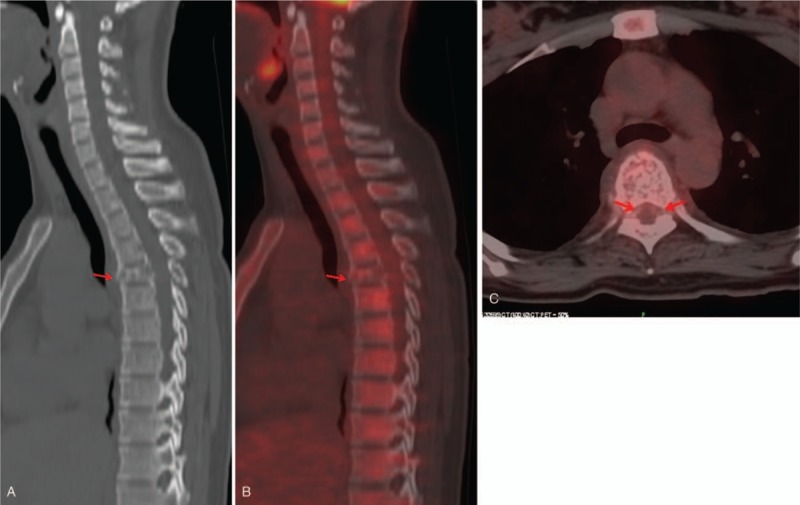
(A) Computed tomography (CT) shows thickenedvertebral trabeculae within theT5 vertebral body.(B) Positron emission tomography–CT (PET-CT) shows milduptakein the T5 vertebral body.(C) PET-CT shows no uptake in the extraosseous components of the lesion.
